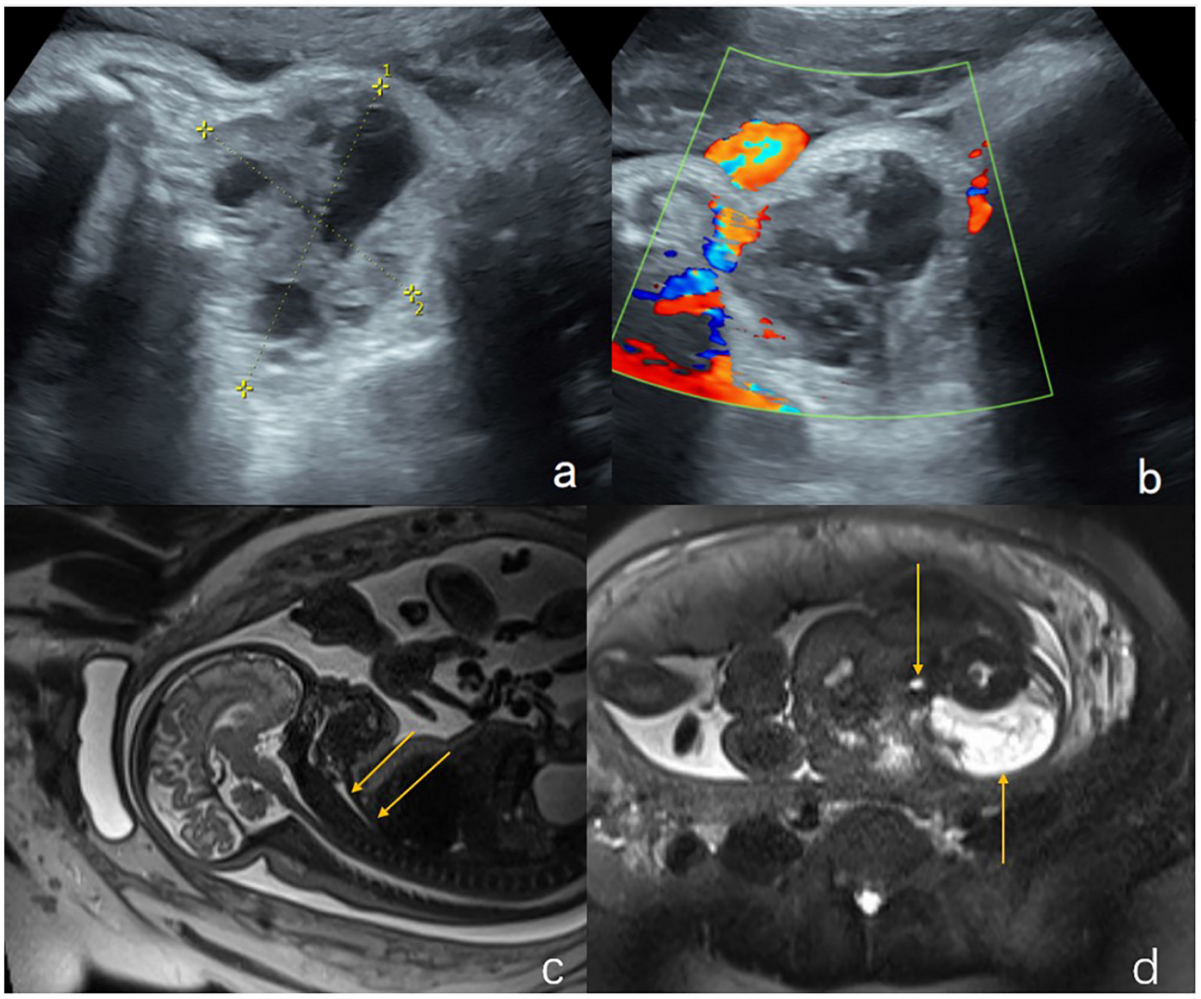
Lymphangioma in the neck of a fetus (case 10). (a,b) Sagittal and axial views of the cervical mass by two-dimensional ultrasonography. A mass on the lateral aspect of the fetal neck (5 cm × 4.4 cm × 4 cm) was found at 25 weeks of gestation. (c,d) Sagittal and axial T2-weighted showed a lobulated heterogenous mass at left neck of the fetus which is hyperintense with hypointense separation within it. Magnetic resonance imaging showed that the left neck of the fetus had a lumpy-long T2 signal that was not uniform and had low signal separation within it. The maximum cross-section of the sagittal position was 5.8 cm × 4.0 cm, the pharyngeal cavity structure was visible, and the trachea was visible throughout, without obvious stenosis. The cervical mass was 6.7 cm × 5.7 cm × 3.1 cm in the 37th week of gestation. Elective cesarean section was performed at 37 weeks + 3 days of gestation. Tracheal intubation was successful in ex utero intrapartum treatment during delivery. After the operation, the newborn had a favorable outcome and is currently 1 year old with normal height, weight, and intelligence.
